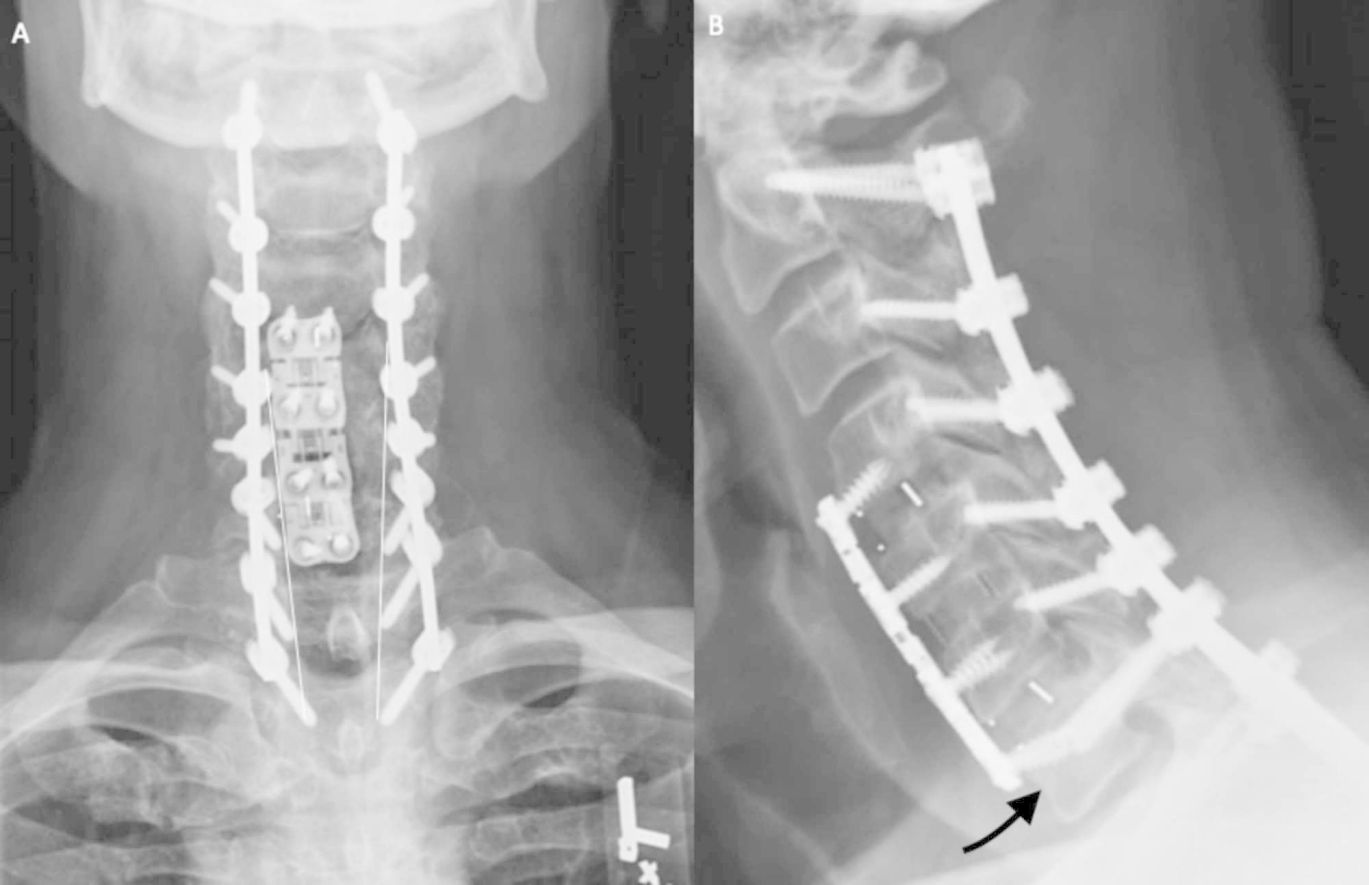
Radiograph breach assessment. (A) The harmonious line conceived by linking the pedicle boundaries at adjacent levels (white lines) allows for assessment of medial and lateral breaches on plain films as previously described by Kim et al. on plain radiographs. (B) Lateral radiographs allow for assessment of anterior breaches by locating the tip of the pedicle screw in relation to the anterior cortex (black arrow).
